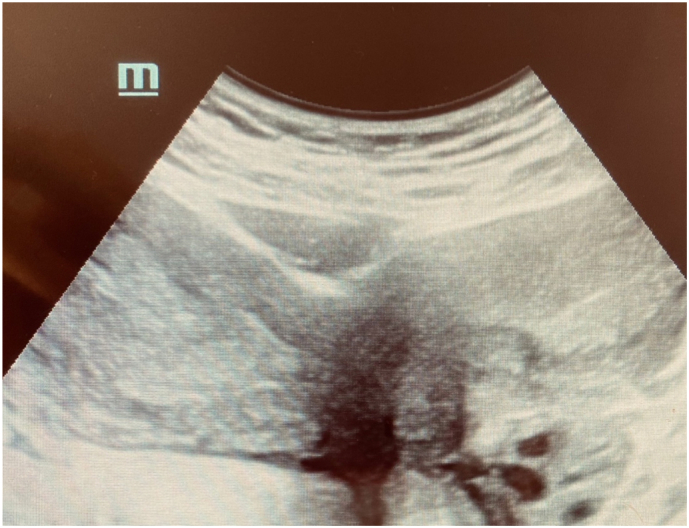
an image of ultrasound showing the uterus didelphys 3 months after surgery.
